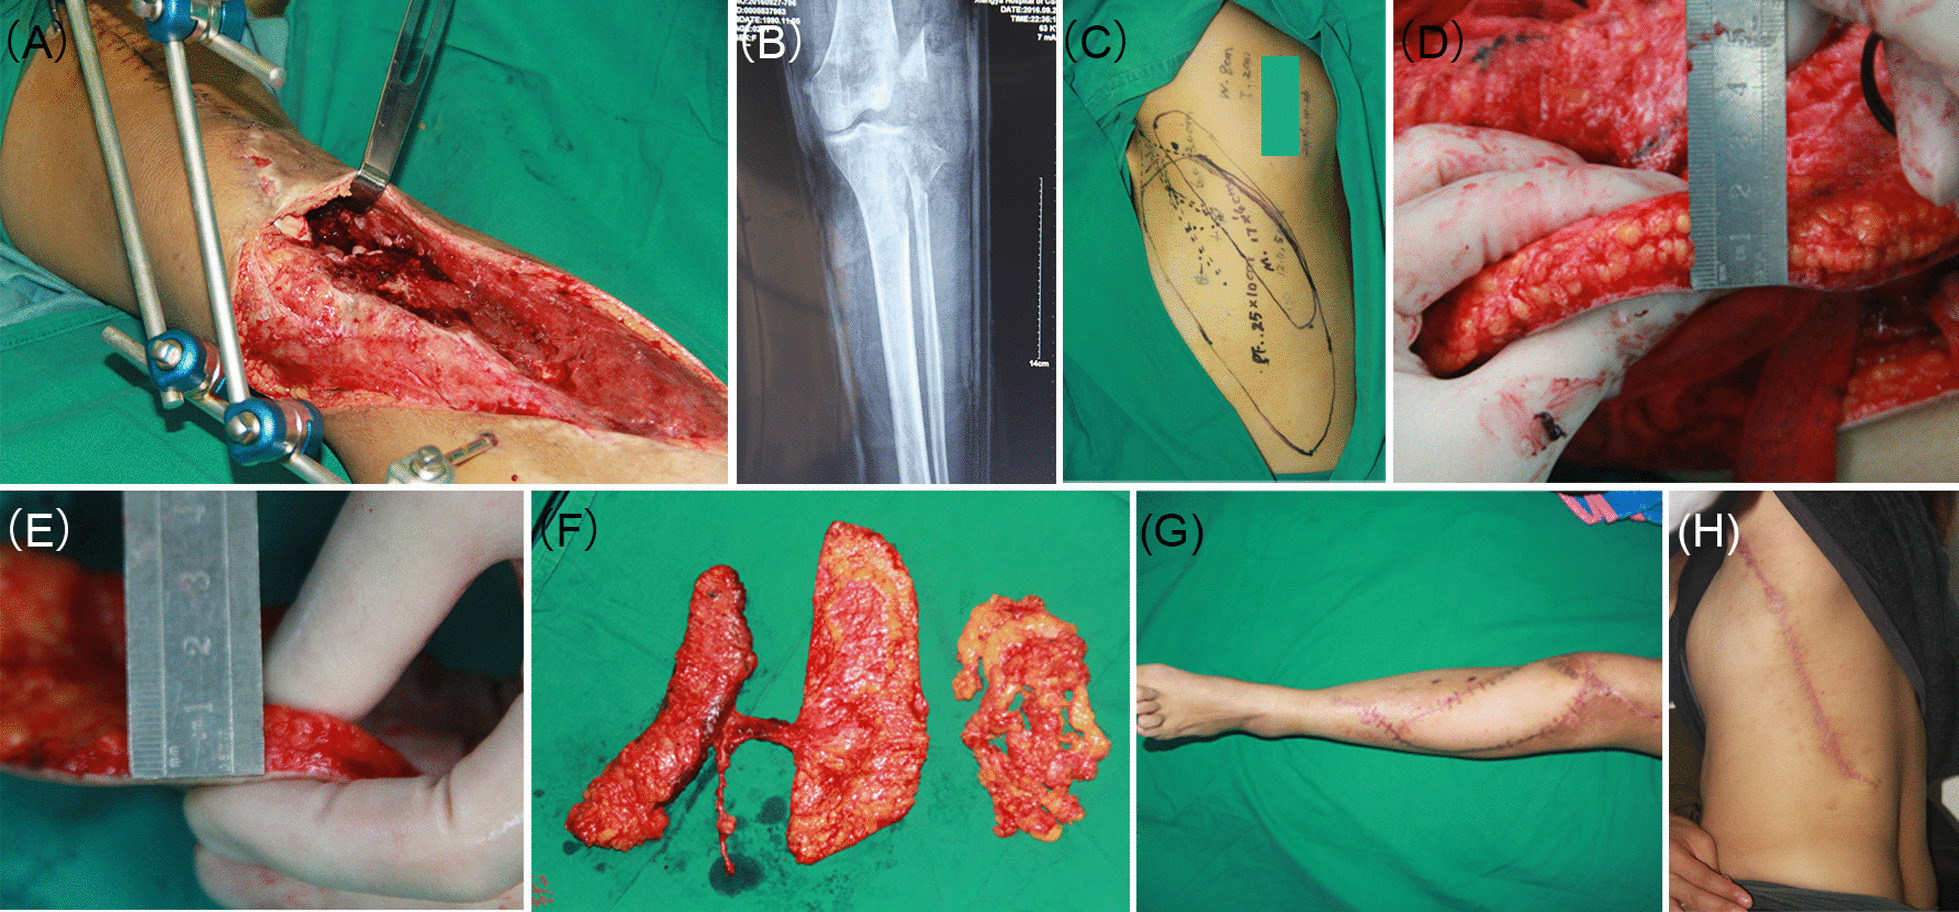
A Large skin defect with deep dead space after debridement and external fixation of the fractures. B Preoperative X-ray photograph. C Flap design of a chimeric and microdissected debulking TDAP flap. D, E Flap thickness before and after microdissected debulking. F The harvested chimeric and microdissected debulking TDAP flap. G, H Postoperative outcomes of the recipient and donor site at 5-month follow-up
